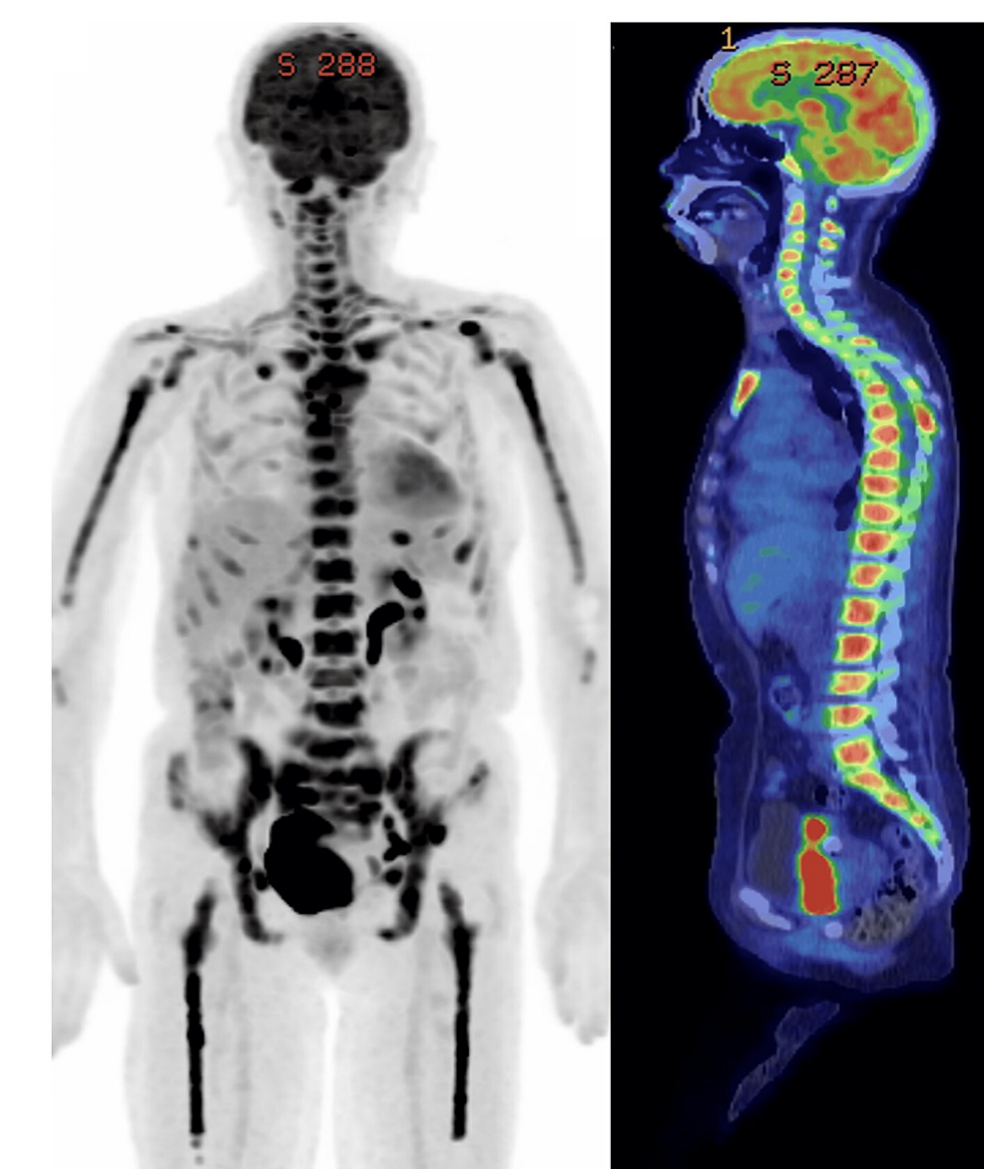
PET-CT showing diffuse fluorodeoxyglucose accumulation in almost all bones in the body PET-CT: Positron emission tomography-computed tomography
